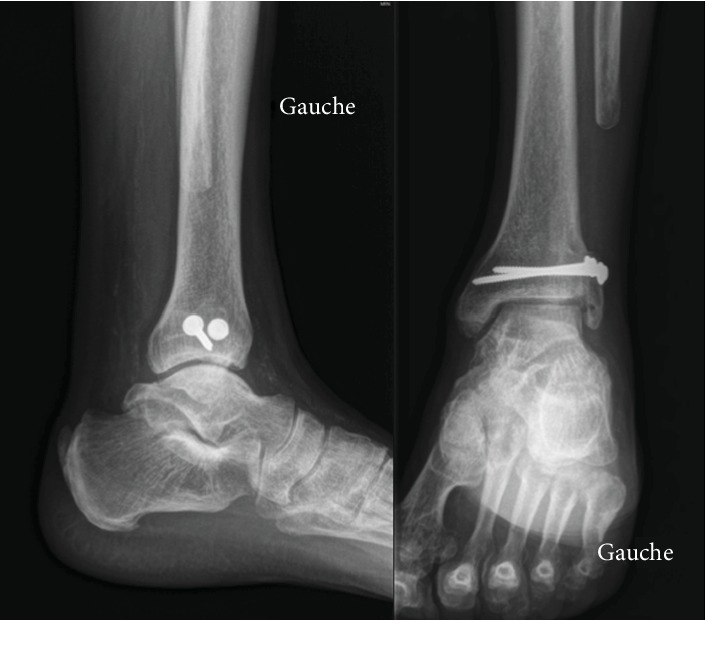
One-year postoperative radiography.
